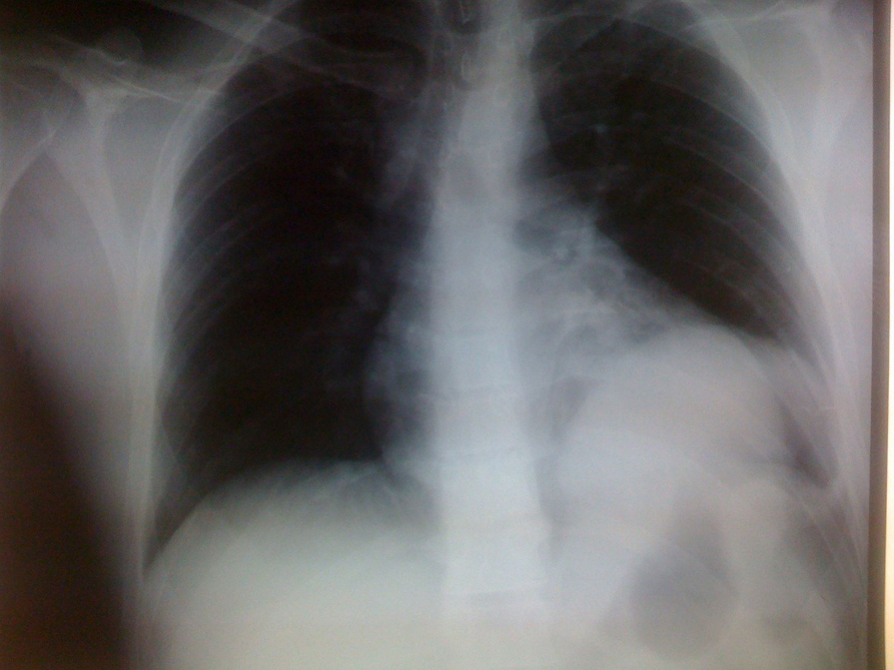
Plain chest x-ray with the stomach in the left hemi-diaphragm.
